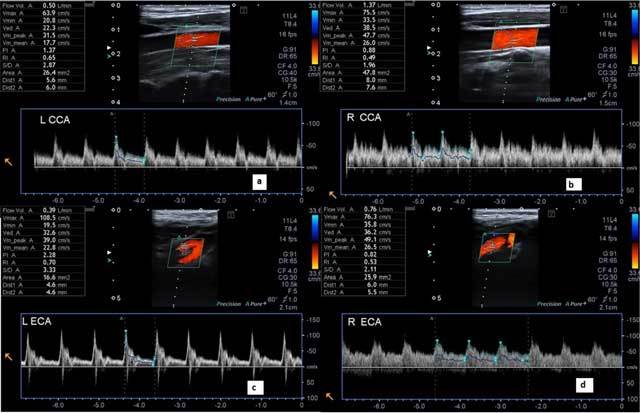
The peak systolic and end diastolic velocities, flow volume rates, and resistive indices of right (a) and left (b) carotid arteries and right (c) and left (d) external carotid arteries were determined with the carotid Doppler sonography.
